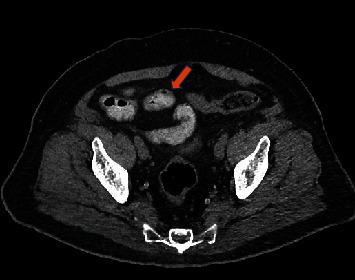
CT scan depicting focal wall thickening of the distal ileum and surrounding fat stranding and air loculi along the wall of the distal ileum (arrow).
